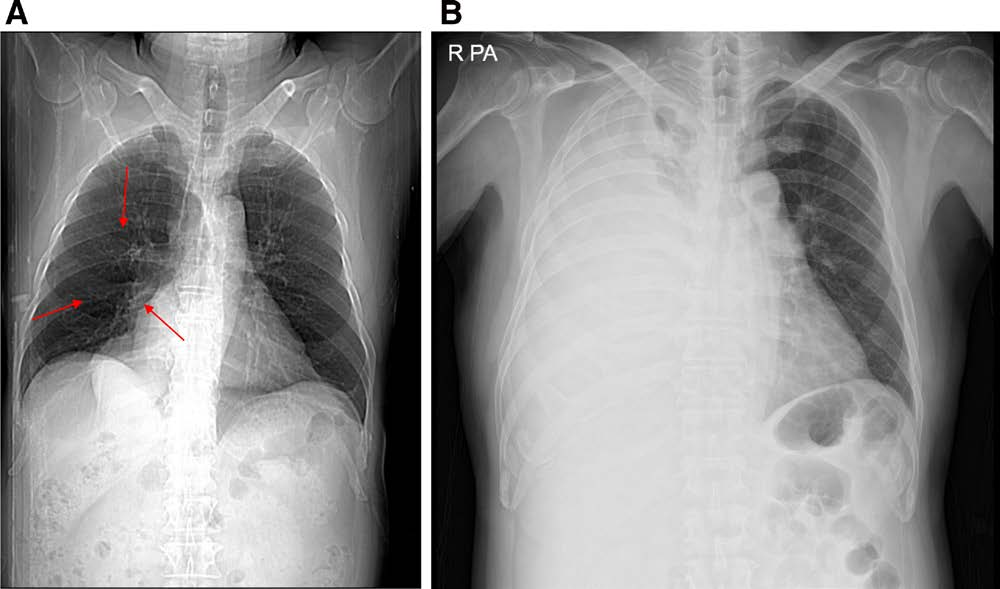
(A) Chest radiograph revealed mass opacities in the right lower lung fields (arrow). (B) Follow-up chest radiography showed a large right pleural effusion with compressive atelectasis.
